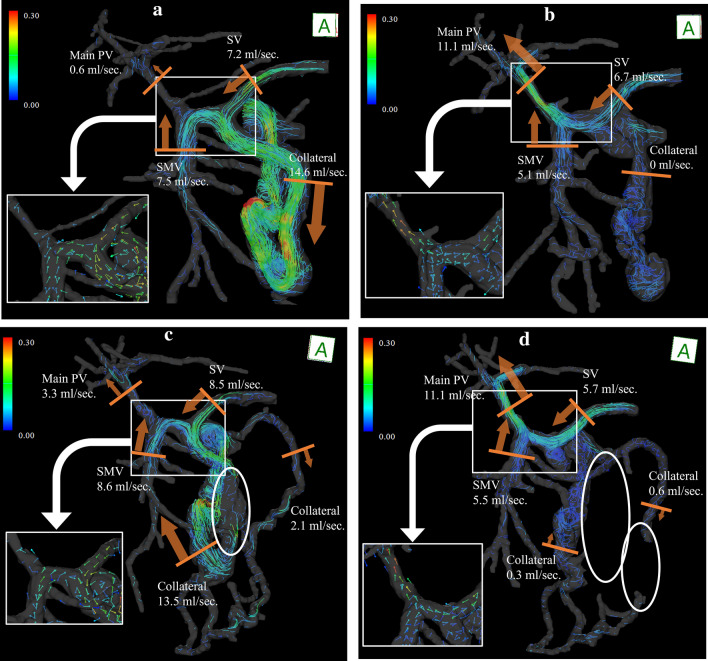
A woman in her 70s presented with cirrhosis and hepatic encephalopathy (HE) associated with large portosystemic shunt (PSS). She also had multiple hepatocellular carcinomas, and transcatheter arterial chemoembolization (TACE) could not be performed owing to portal vein reflux at the previous hospital. a Pre-interventional 4D Flow MRI showed a large PSS between the inferior mesenteric vein and the left ovarian vein, which was flowing from most of the superior mesenteric and splenic venous blood flow; the shunt rate of the superior mesenteric vein (SMV) blood flow was 95%, and the serum ammonia level was elevated to 177 μg/dL. This was considered the main cause of HE. b Post-interventional 4D Flow MRI. Shunt embolization caused an antegrade change in the superior mesenteric and splenic venous blood flow and increased the blood flow in the main portal vein. The serum ammonia level decreased to 55 μg/dL and HE improved. TACE could be safely performed. c Pre-re-interventional 4D Flow MRI. Three months after shunt embolization, the serum ammonia level again increased to 199 μg/dL. The coil embolization site (circle) has lost signal owing to metallic artifacts. However, a large amount of blood flow signal is seen in the PSS again, suggesting reopening. The shunt rate of the SMV was 62%. In addition, a new hepatofugal colonic marginal vein had developed. Portal vein blood flow was reduced, and shunt re-occlusion was performed. d Post-re-interventional 4D Flow MRI. Coil embolization area (circle) lost signal. Blood flow in the shunt almost disappeared, and blood flow in the main portal vein increased again. The serum ammonia level improved to 58 μg/dL. Following TACE sessions could be safely performed, and there was no recurrence of HE. *PV*, portal vein; *SMV*, superior mesenteric vein; *SV*, splenic vein
